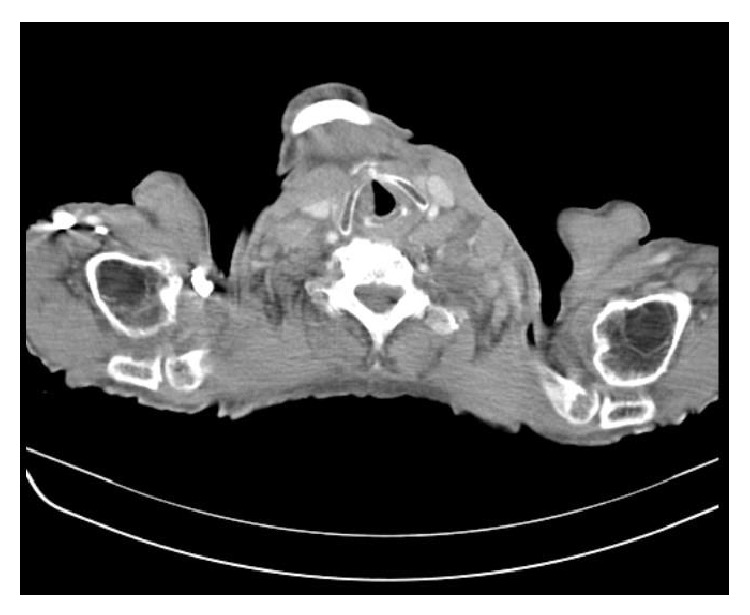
Cervical CT demonstrating multiple enlarged lymph nodes in the paratracheal, subcarinal, bilateral supraclavicular, and cervical regions.
